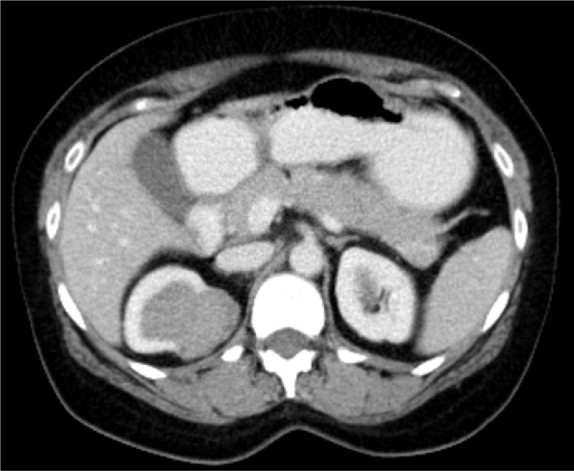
Contrast-enhanced CT scan of the abdomen showing renal mass in the right kidney.
